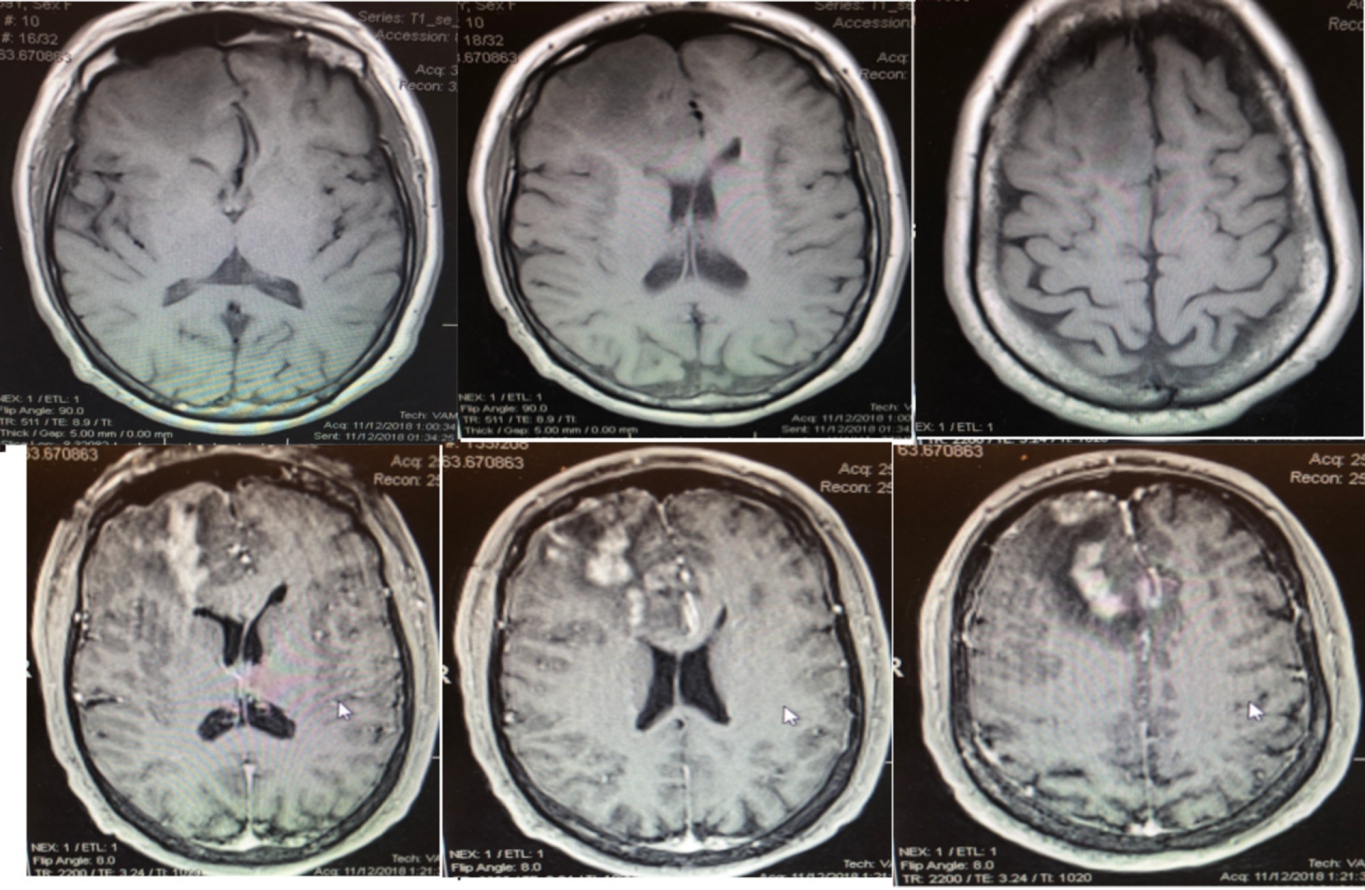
MRI with and without contrast showing large right frontal heterogeneously enhancing mass with surrounding vasogenic edema and mass affect on right lateral ventricle. Top Row: T1 axial without contrast Bottom Row: Axial T1 with contrast
